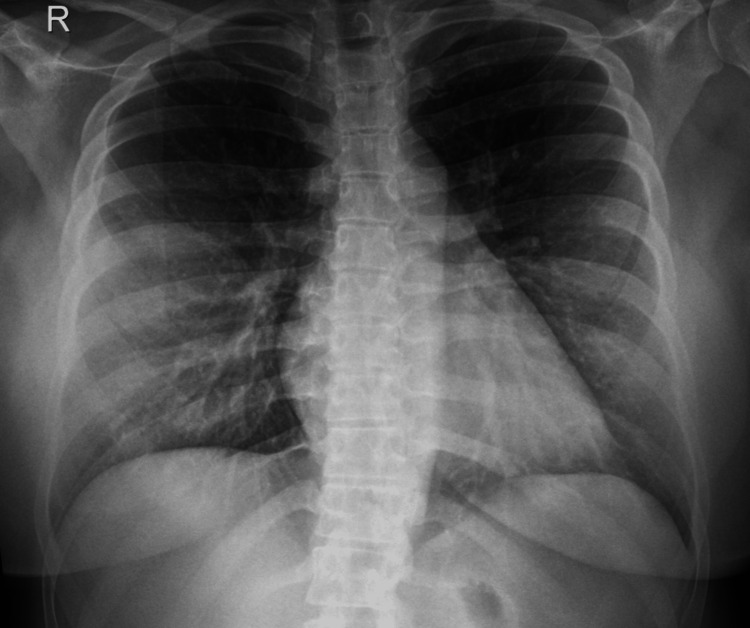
Pre-operative chest X-ray with unremarkable findings
